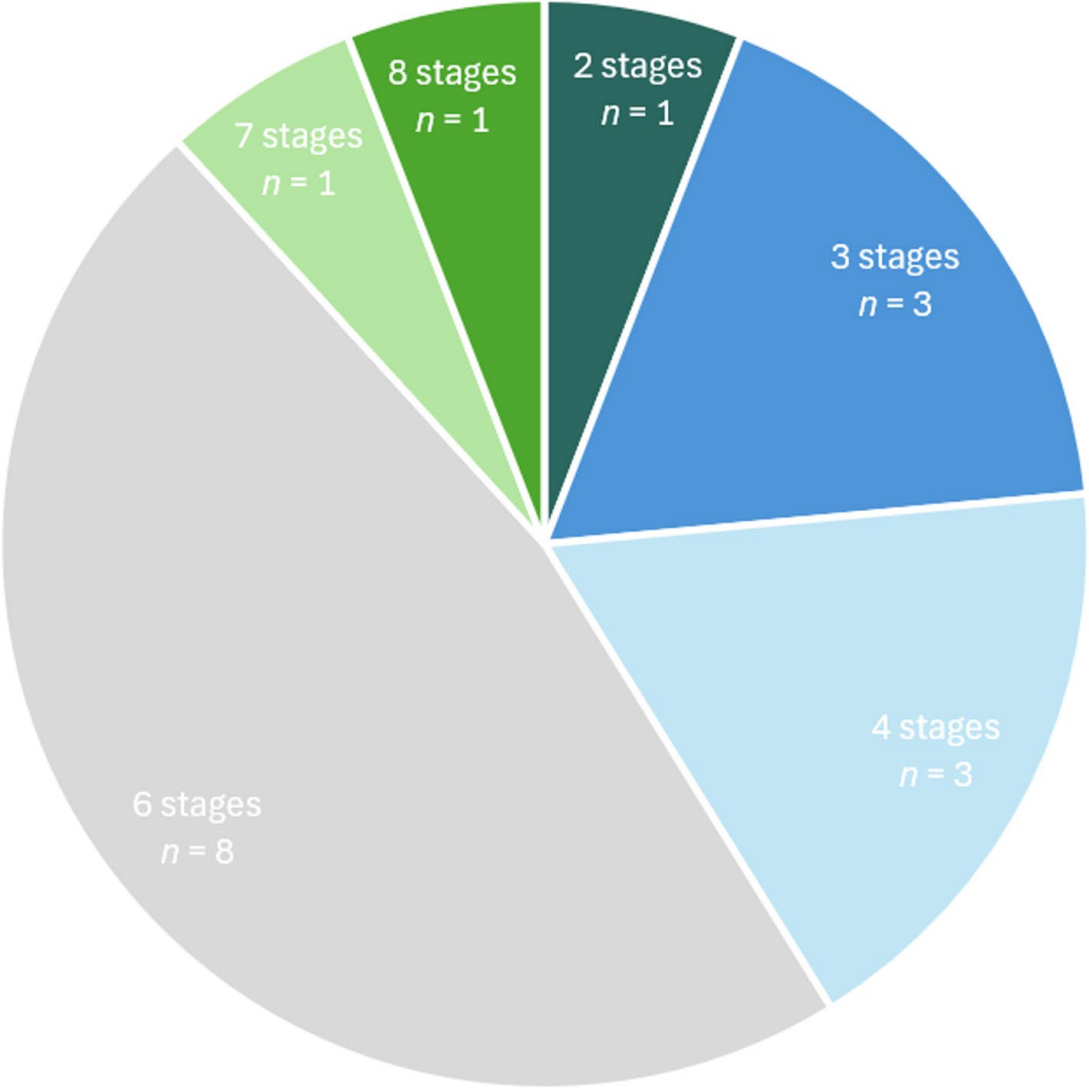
Pie chart showing the number of healing stages assessed to estimate fracture age across the 17 papers using 2D x-rays. *N* numbers represent the number of papers using the specified number of stages
